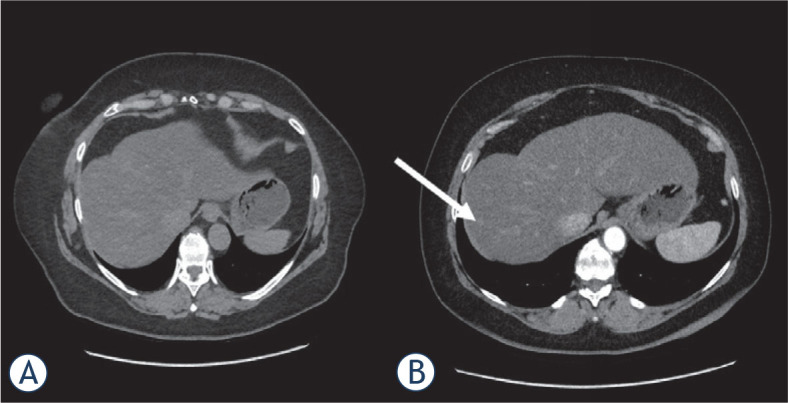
A male patient with a suspect and unclear hepatic lesion with 6 mm in segment 7. While not visible in the unenhanced CT (A), the lesion impressed arterial hypervascularized after intravenous contrast medium application (B) (white arrow).
